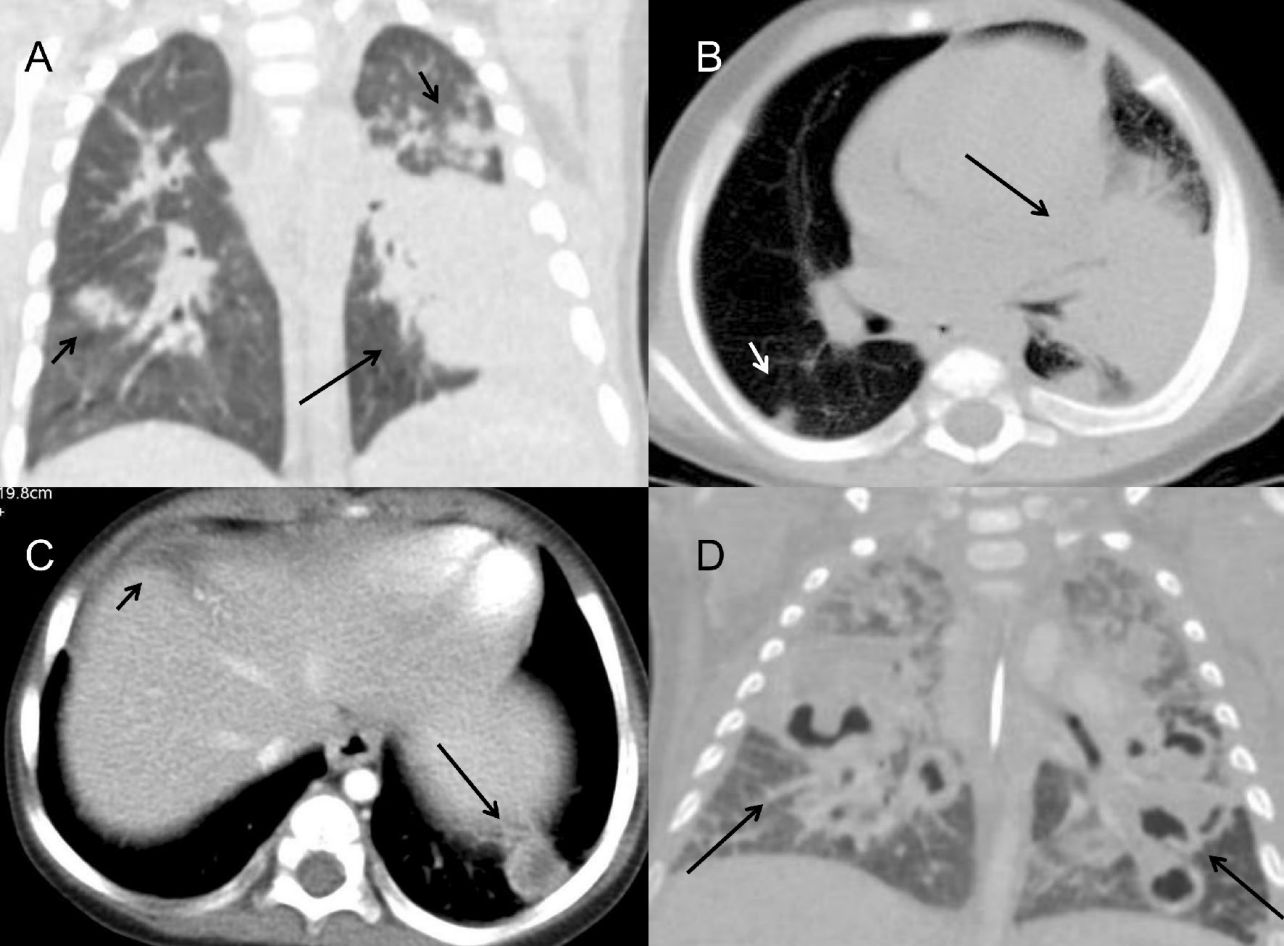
Bacterial infection in CGD patients. (A,B) A 5-month-old boy with klebsiella pneumoniae infection showed a large area of consolidation in the left lower lobe on axial and coronal reformat CT images (long arrow). Multiple nodular lesions in the bilateral lung fields were found (short arrow). (C) A 1-year-old boy with Staphylococcus aureus infection. Chest CT showed the small nodular lesion with abscess inside in left lower lobe (long arrow). The low-attenuated lesion in the left lobe of liver was seen, suggesting the possibility of hepatic abscess (short arrow). (D) A 1-year-old boy with Staphylococcus aureus infection. On CT, masses and patchy consolidations were found in the bilateral lung fields with multiple small cavities formation (long arrow).
